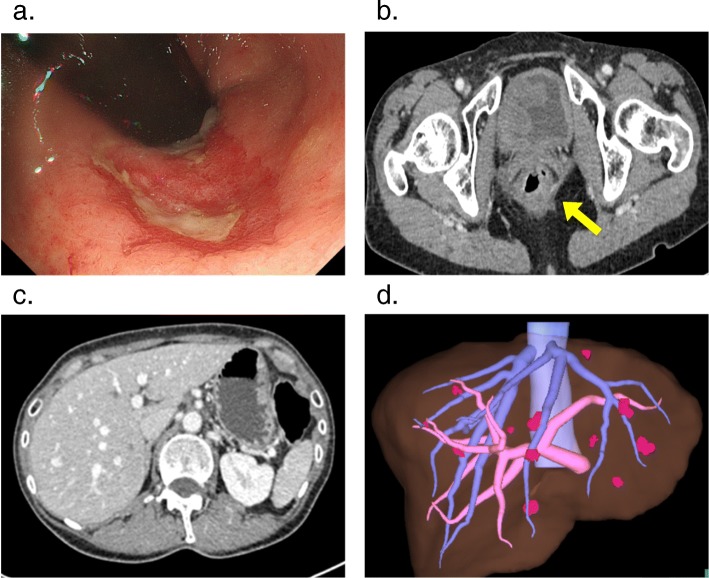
CT imaging and a colonoscopy after neoadjuvant chemotherapy. a Primary rectal cancer on a colonoscopy. The rectal cancer became flat and exceedingly shrunken. b Axial view of the primary CRC (arrow) on a CT. Contrast-enhanced wall-thickening (13mm) has been improved. c Axial view of multiple bilobular liver metastases on CT. The metastatic lesions shrunk prominently. d The 3-dimensional image of the multiple bilobular liver metastases. All metastatic lesions shrunk prominently. The estimated remnant liver volume was 73.4%. CRC, colorectal cancer; CT, computed tomography
